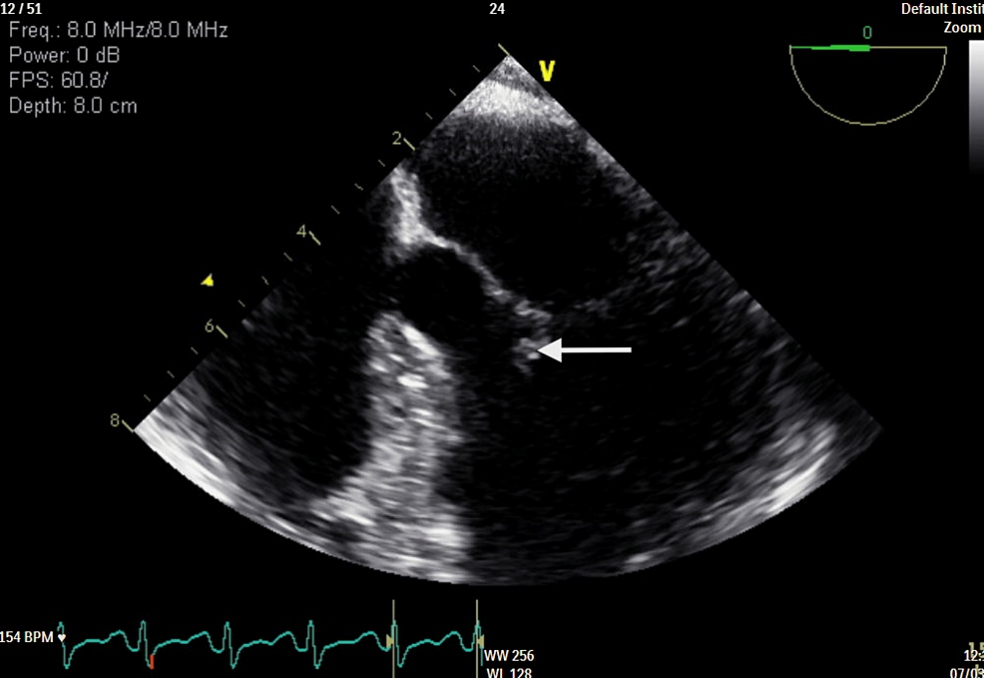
Transesophageal echocardiogram showing vegetation on the anterior leaflet of the mitral valve
